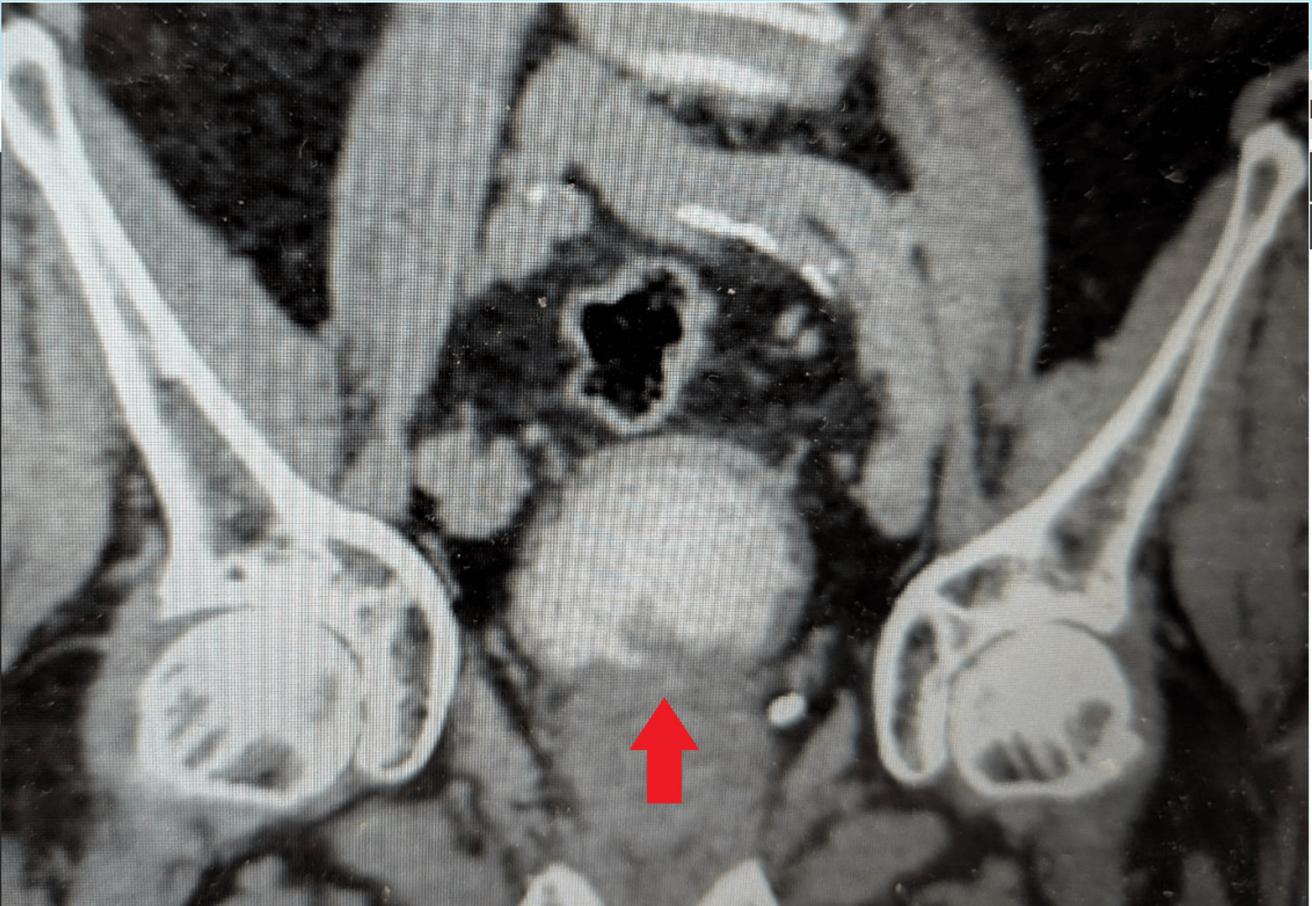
CT urogram showing small bladder filling defect (Case 3) A well-circumscribed intraluminal filling defect is seen within the urinary bladder (red arrow), compatible with a polypoid lesion. No upstream hydroureteronephrosis or additional pelvic abnormalities are evident on this slice, concordant with a solitary bladder lesion later confirmed endoscopically.
